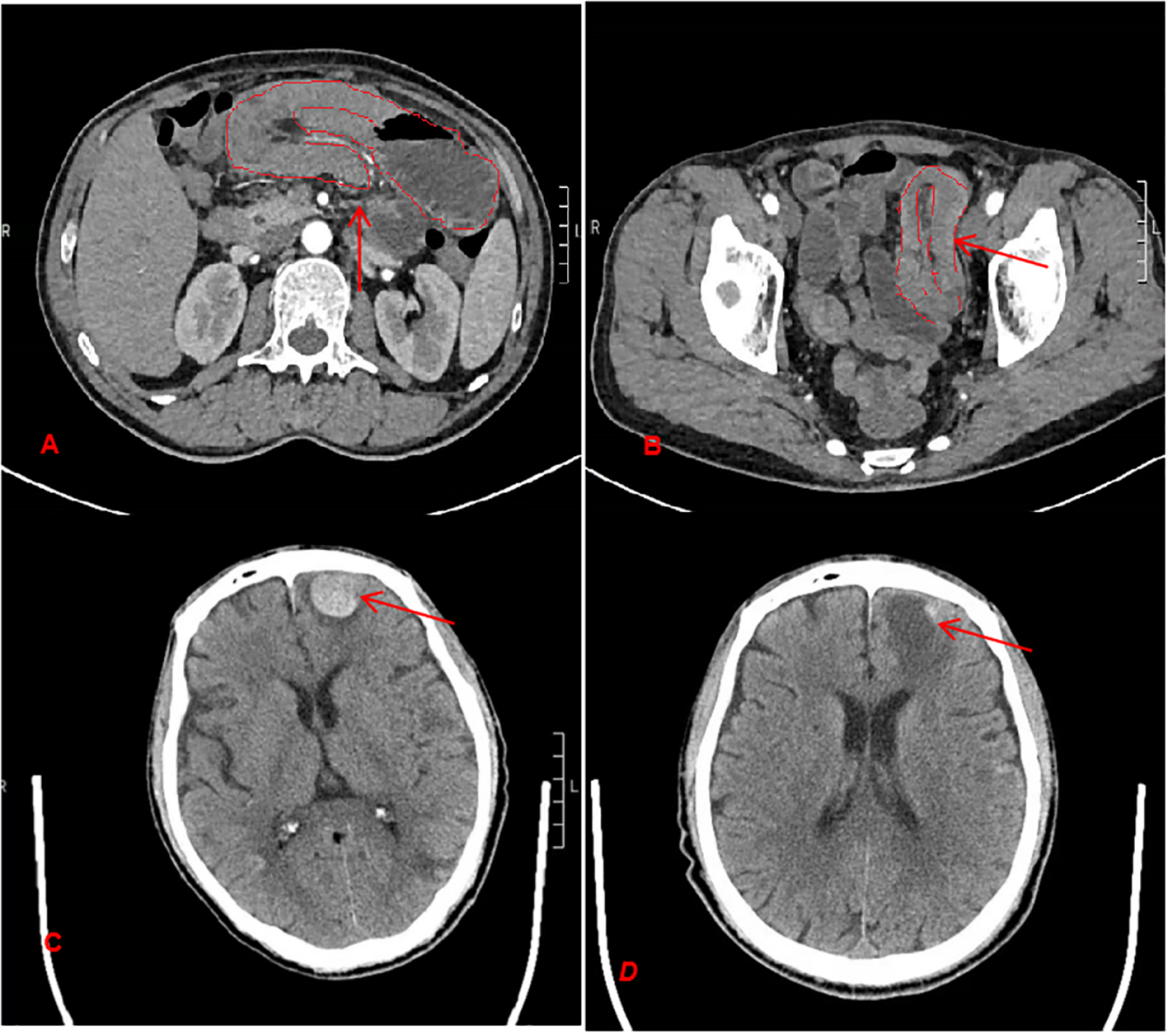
CT findings of small bowel intussusception and intracranial metastases. (A, B) Contrast-enhanced abdominal CT scans showing small bowel intussusception. The arrows indicate the characteristics target sign (A) and the intussusception bowel loop at the site of intussusception (B, C) Pre-treatment head CT showing a metastatic lesion in the left parietal lobe, with local hyperdensity (arrow). (D) Post-radiotherapy head CT demonstrating near-complete resolution of the previously seen metastasis (arrow), with mild post-treatment edema in the adjacent brain parenchyma.
